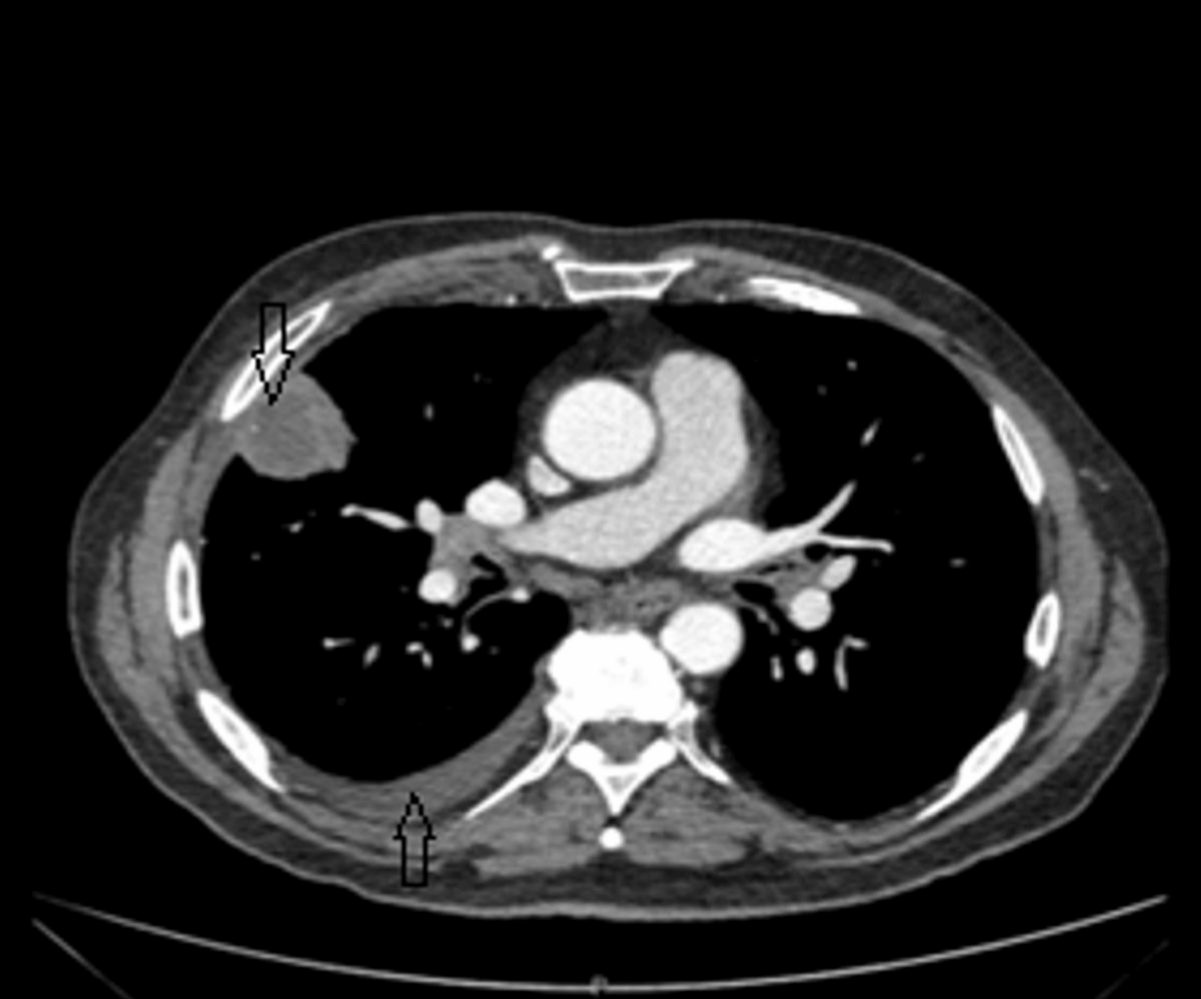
The CT scan of the chest's mediastinal window showed a pleural-based, low-density mass in the periphery of the middle lobe, measuring 38 × 36 mm, and small right-sided pleural effusion.
